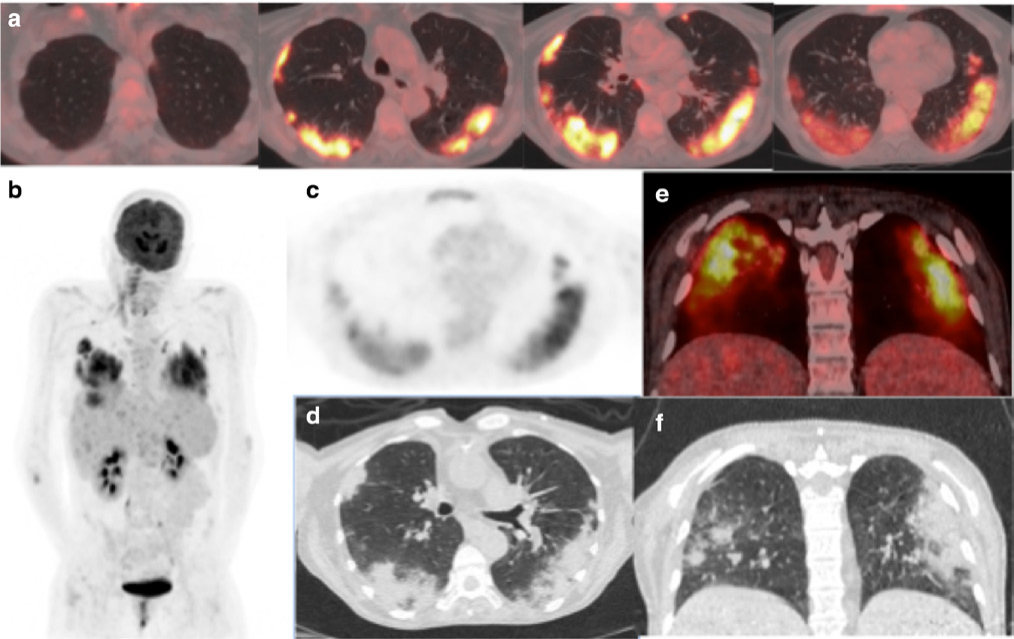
(a) Axial-fused thoracic ^18^FDG-PET/CT, (b) maximum-intensity projection, (c) ^18^FDG-PET thorax, (d) axial low-dose CT thorax (e) coronal fused ^18^FDG-PET/CT, (f) coronal thoracic CT. The images demonstrate hypermetabolic patchy peripheral consolidation and GGO in four lobes and subcarinal lymph node. ^18^FDG-PET, 18-fludeoxyglucose-positron emmision tomography; GGO, ground glass opacity.
